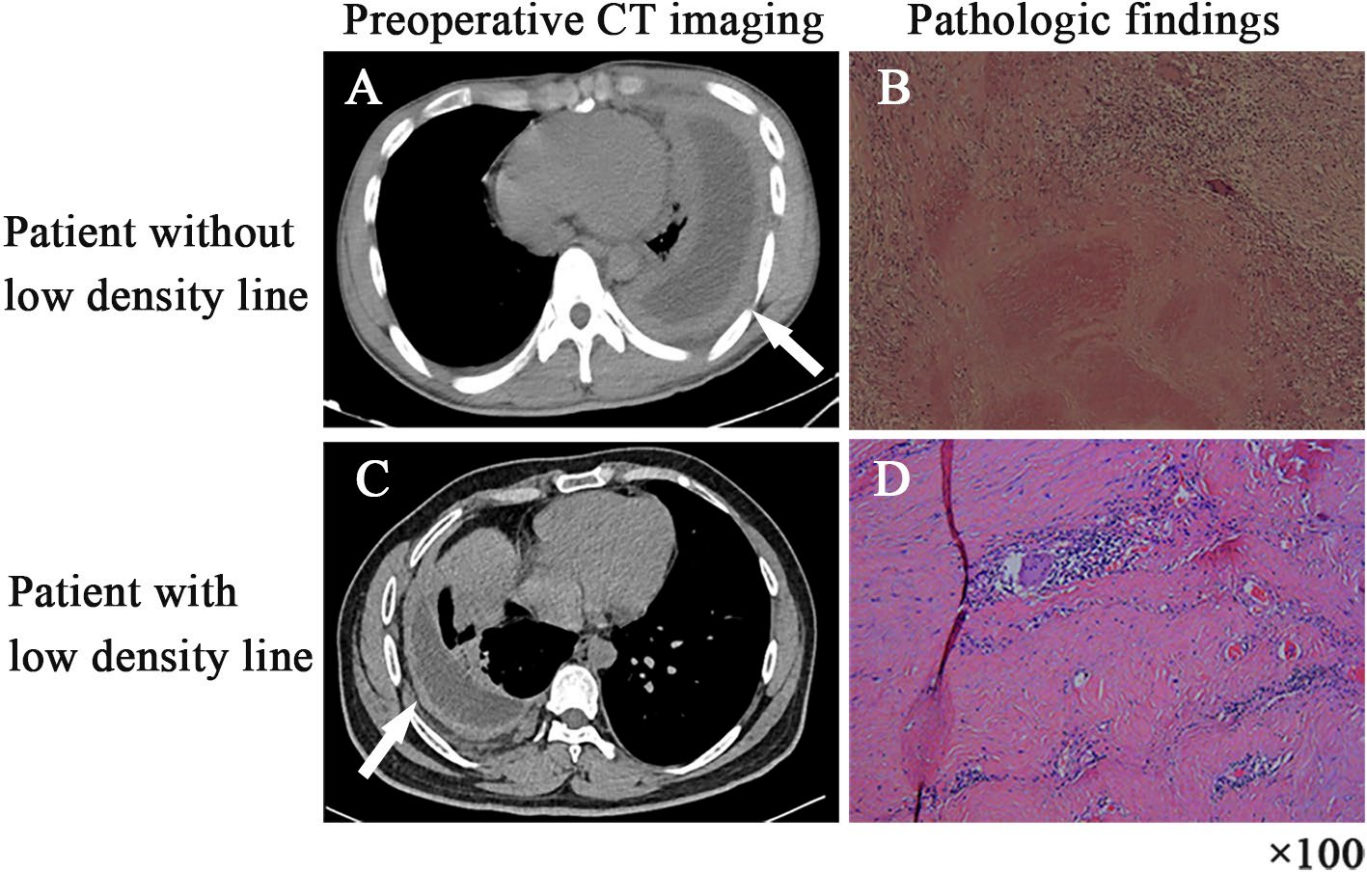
CT and pathological pictures of patients with or without low-density lines (A). There was no obvious boundary between the fibrous pleural rind and the parietal pleura (B). The typical pathological change in patients without a low-density line was caseous necrosis (C). The distinct “low-density line between the fibrous pleural rind and the parietal pleura” (D) The typical pathological change in patients with a low-density line was hyperplasia with hyaline degeneration.
